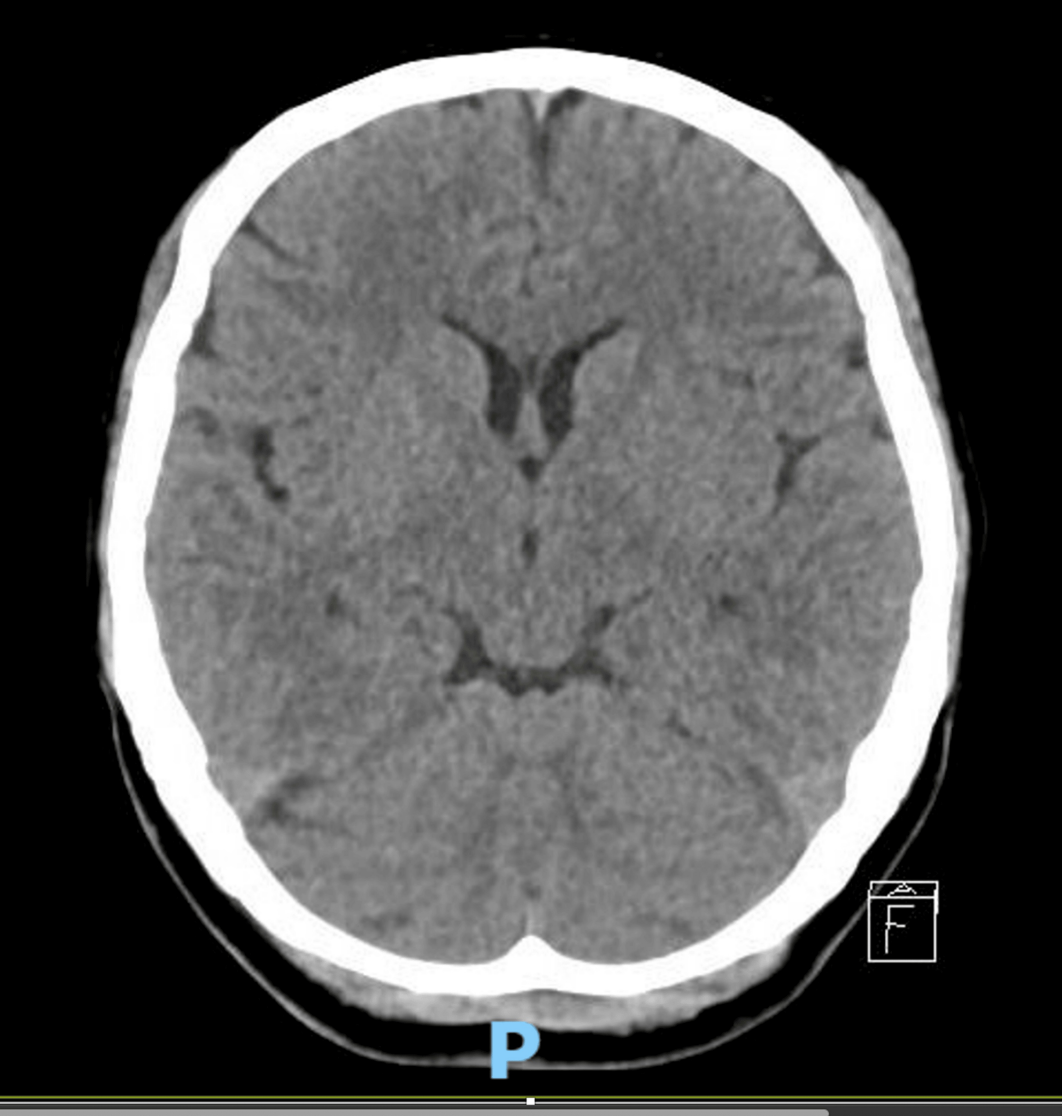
Noncontrasted CT brain revealed no evidence of acute intracranial abnormalities such as infarct, hemorrhage, or mass effect. The ventricular system appeared normal in size and configuration CT: computed tomography
